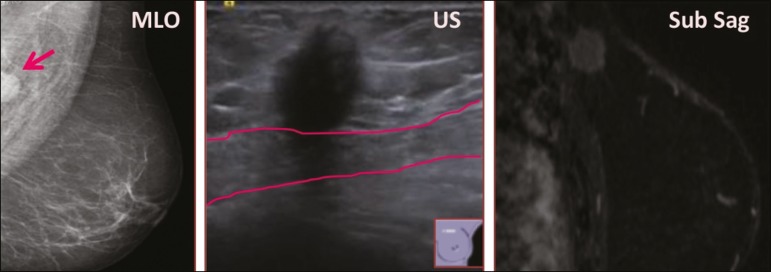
Digital mammography (mediolateral oblique view), ultrasound, and MRI in a 54-year-old woman (case 2) with a pathologically confirmed GCT in the upper inner quadrant of the left breast. The location of the mass was deep in the tissue, adjacent to the pectoralis muscle, which is partially intercepted in the mediolateral oblique view. However, it is so medially located that it is not intercepted in the craniocaudal view (not shown). On ultrasound, the mass showed suspicious features, such as nonparallel orientation (relative to the chest wall), microlobulated contours, and posterior acoustic shadowing. Note the close proximity to the pectoralis muscle (lined in pink), suggesting that the mass is adherent to it. The fine spiculated margins are best appreciated in the subtracted sagittal image.
